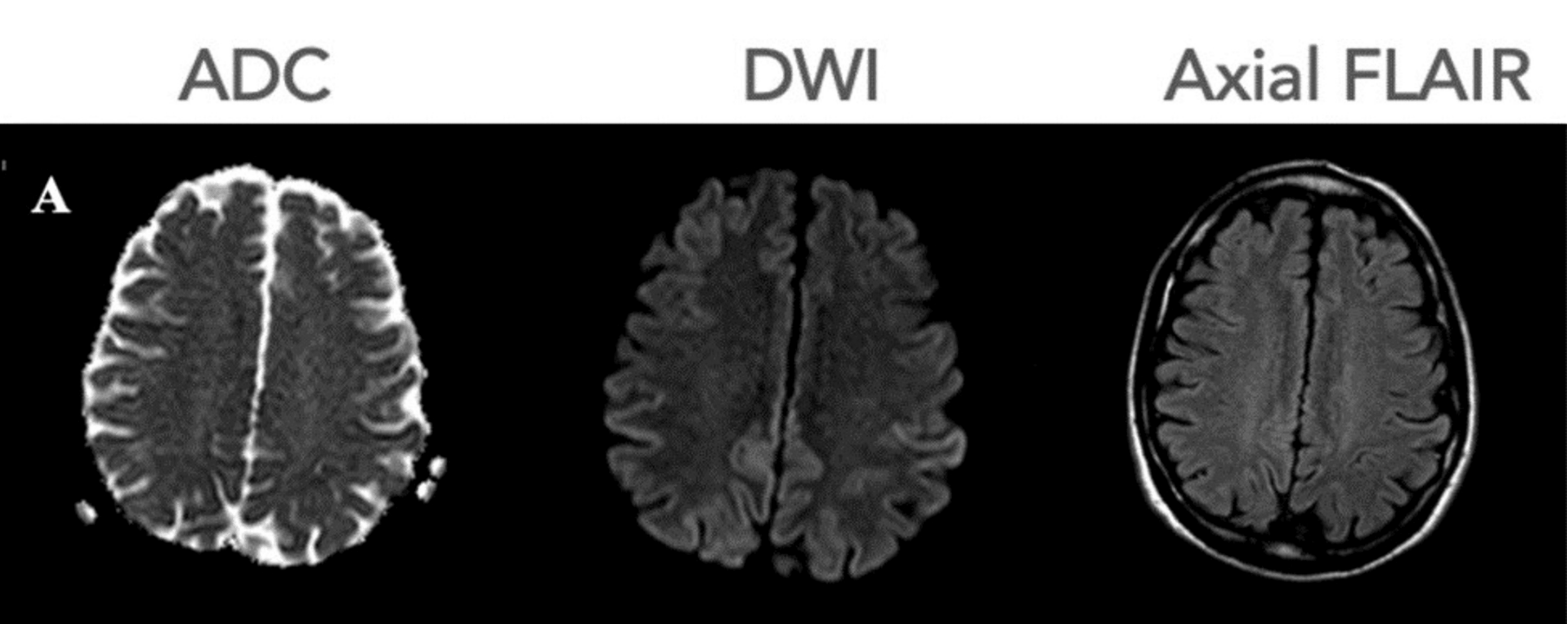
Brain magnetic resonance imaging (MRI) at the patient’s first visit (September 22, 2022) showing subtle abnormal signal intensity throughout most of the parietal cortex bilaterally. Some of these areas were associated with subtle restricted diffusion. ADC: apparent diffusion coefficient map; DWI: diffusion-weighted image; FLAIR: fluid attenuated inversion recovery
